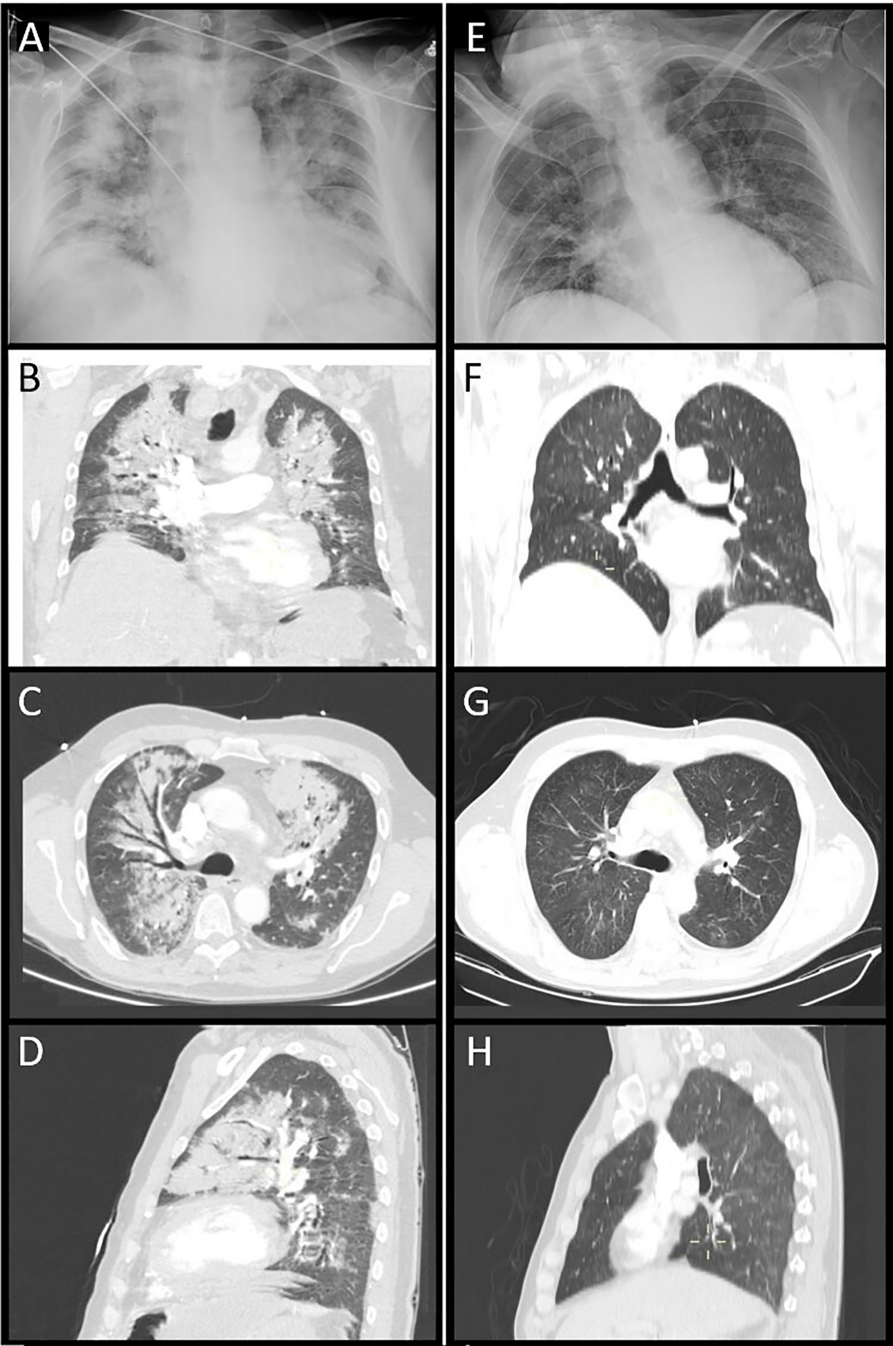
Thorax radiography (A) and CT images [coronal view, (B); axial view, (C); sagittal view, (D)]. Diffuse bilateral patched consolidations with open bronchi inside and ground-glass opacities due to COVID-19 infection. Thorax radiography (E) and CT control images. Two weeks after treatment [coronal view, (F); axial view, (G); sagittal view, (H)], significant improvement of the pneumonia is observed.
